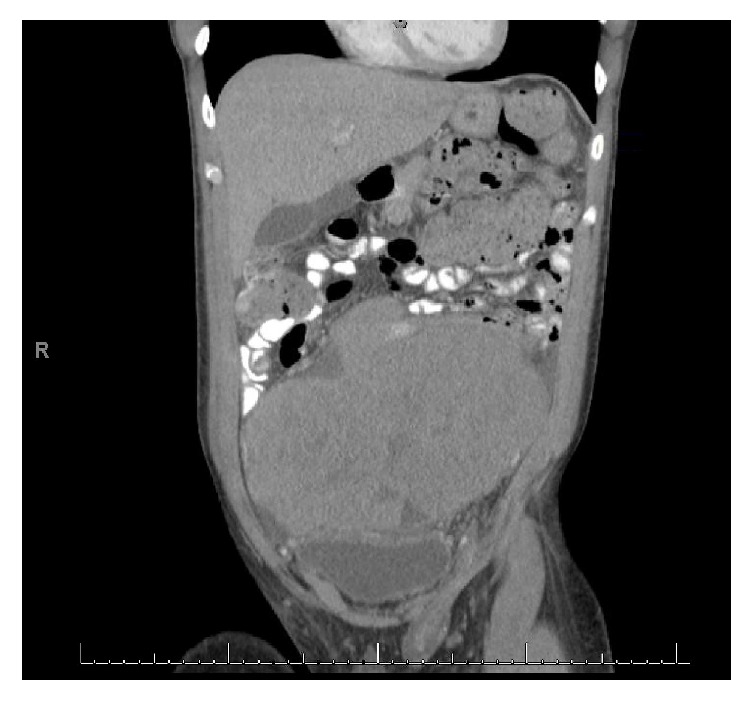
Coronal CT showing mass discreet from undescended left testicle.
